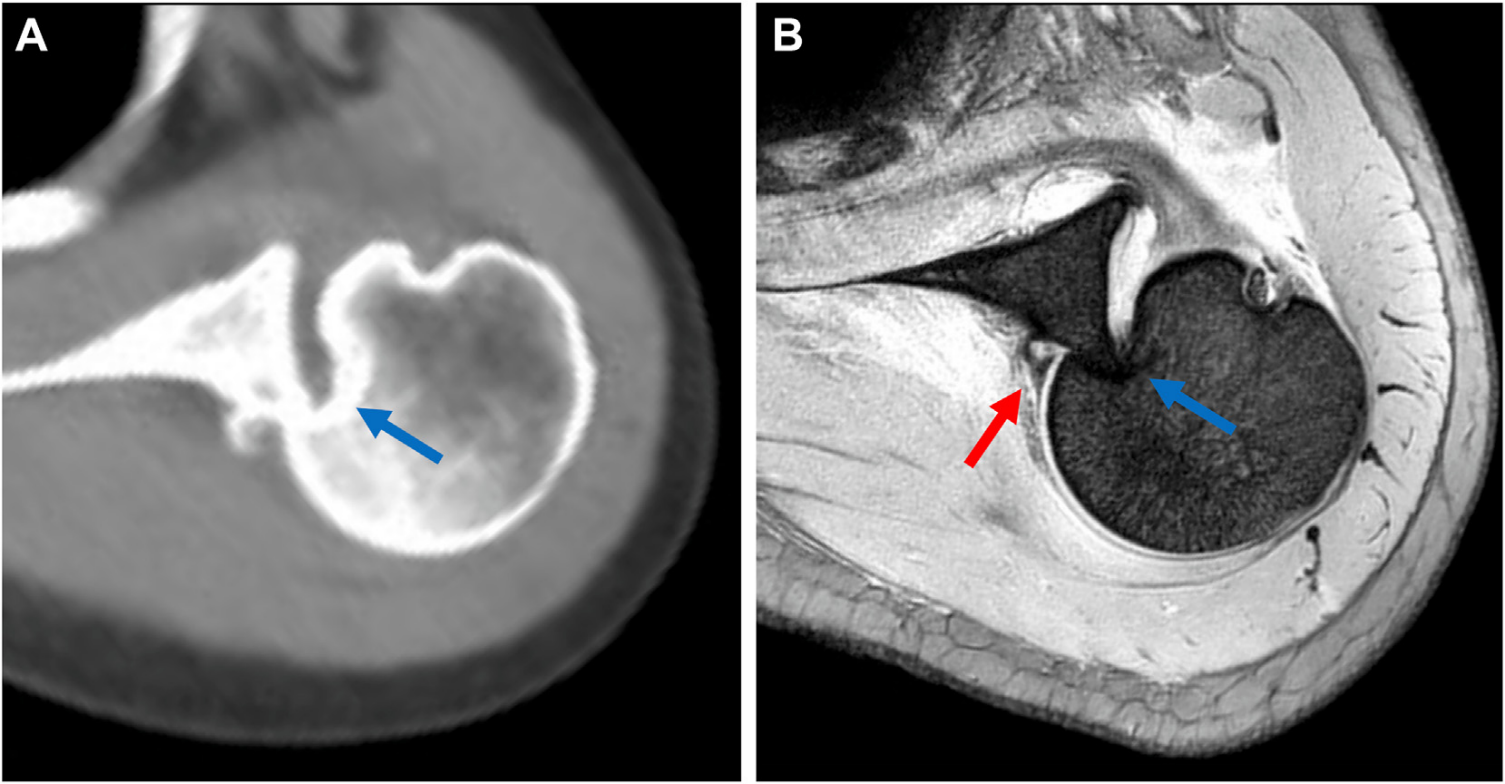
(A) CT and (B) T2 star–weighted MRI images of Case 1, obtained at a local hospital, revealed a Hill-Sachs lesion (*blue arrow*) and a posterior Bankart lesion (*red arrow*). *CT*, computed tomography; *MRI*, magnetic resonance imaging.
